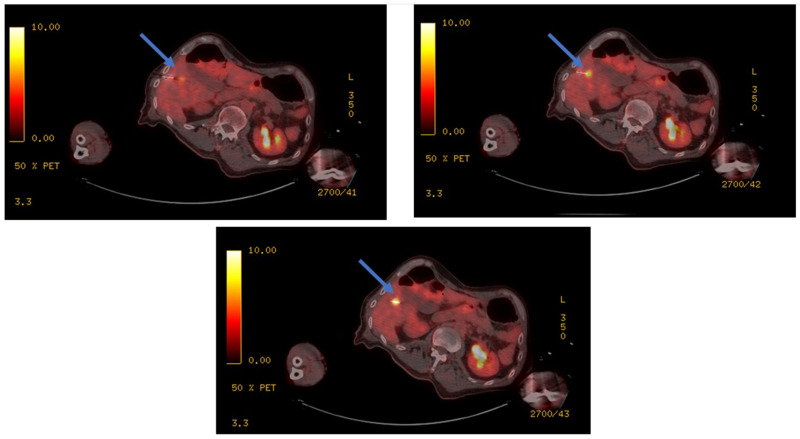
Real-time PET provides the benefit of continuous depiction of the FDG avid CLMs during the ablation and eliminates the need for multiple contrast injections. The location of the tumor and the ablation electrode is depicted with blue arrows in three consecutive PET/CT slices.
